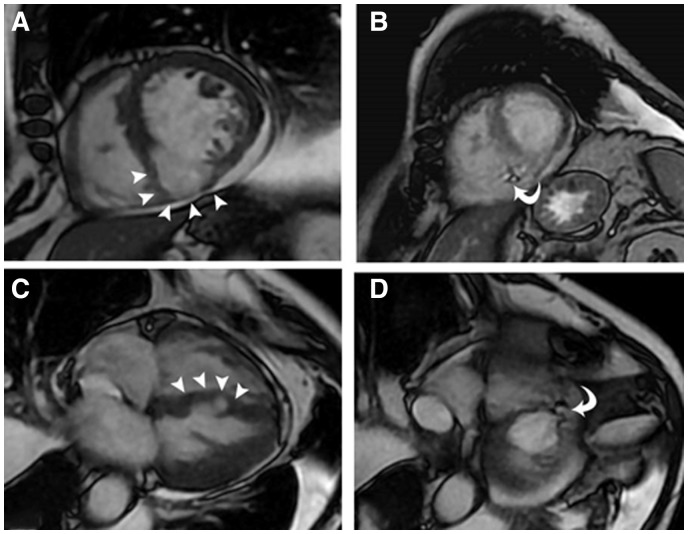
Cardiac magnetic resonance imaging: Balanced steady-state gradient echo cine images [short-axis plane (*A, B*), four-chamber plane (*C, D*)]. The LV shows a mid-inferior and inferoseptal wide-neck -true aneurysm (arrow heads in *A, C*) with a focal area of ventricular septal rupture in the inferior aspect of the aneurysm with a turbulent high-velocity flow across it (curved arrows in *B, D*).
